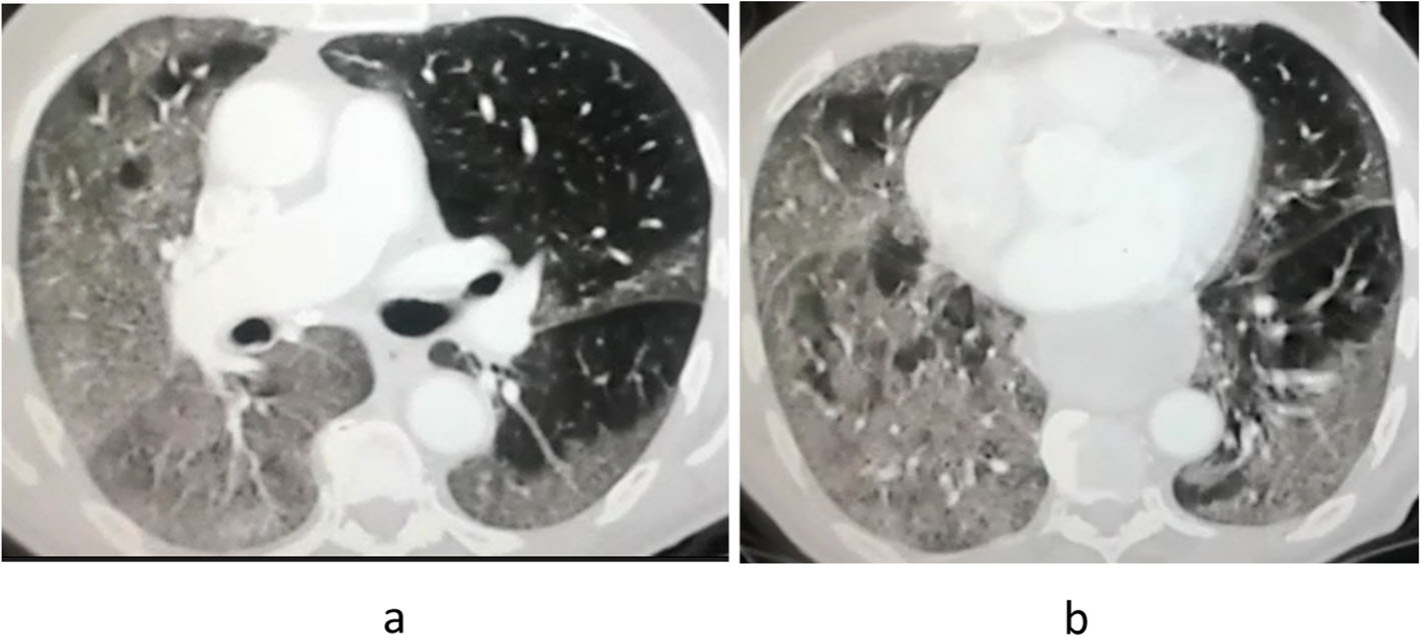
a, b Axial CT chest lung window in a 67-year-old male patient showed a diffuse homogenous ground-glass density of both lungs, more evident on the right side (white lung). Atypical CT features of COVID-19; clinically, this patient suffered from severe clinical symptoms progressed to ARDS and then mechanically ventilated
